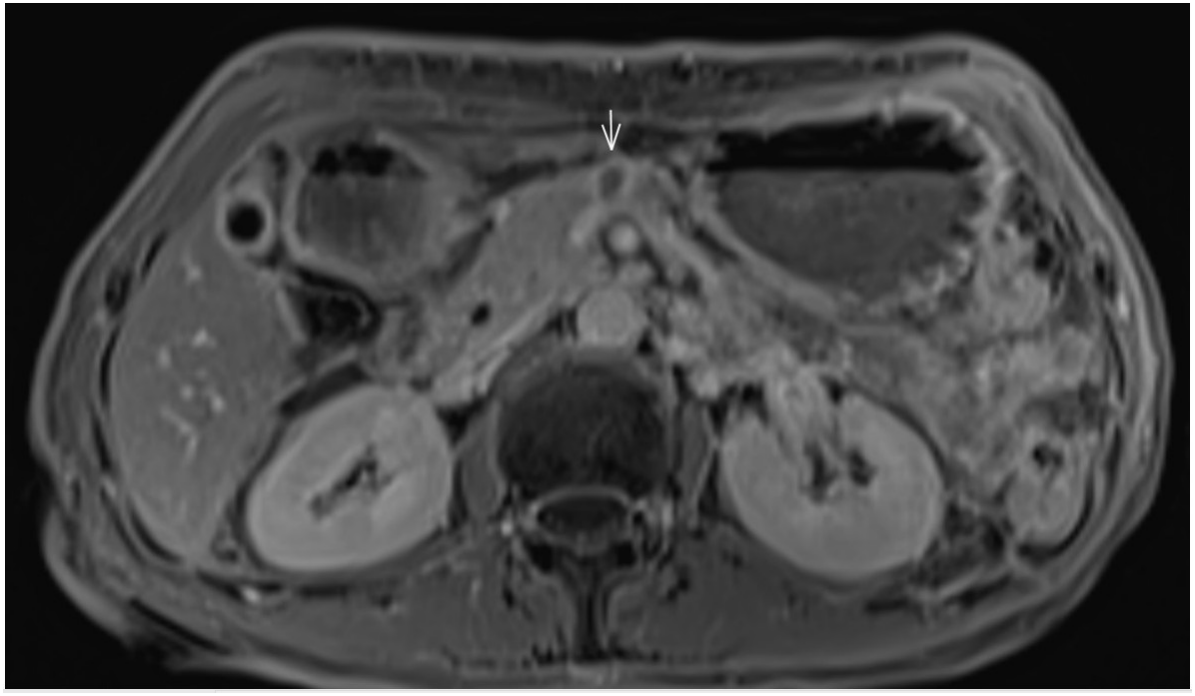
Stabilization of pancreatic mass following SBRT. Axial post-contrast T1-weighted MRI demonstrates a 1 x 1,6 cm focal lesion in the pancreatic body following SBRT, with central necrosis and peripheral signal intensity enhancement, assessed as stable disease.
